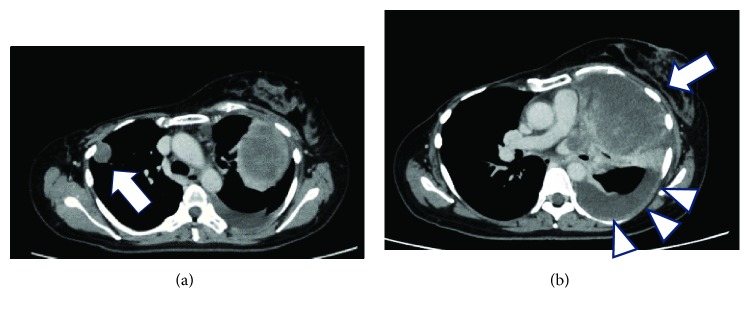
Computed tomography scan acquired before doxorubicin-ifosfamide (AI) chemotherapy showing (a) a 2.2 cm mass in the right lung (arrow) and (b) a 10 cm mass (arrow) and pleural effusion in the left lung (arrowheads).
